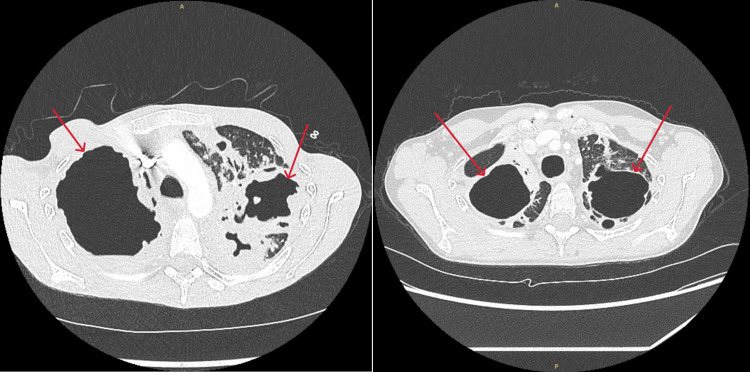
Computed tomography of the chest showing cavities before (left) and after (right) tuberculosis treatment.
